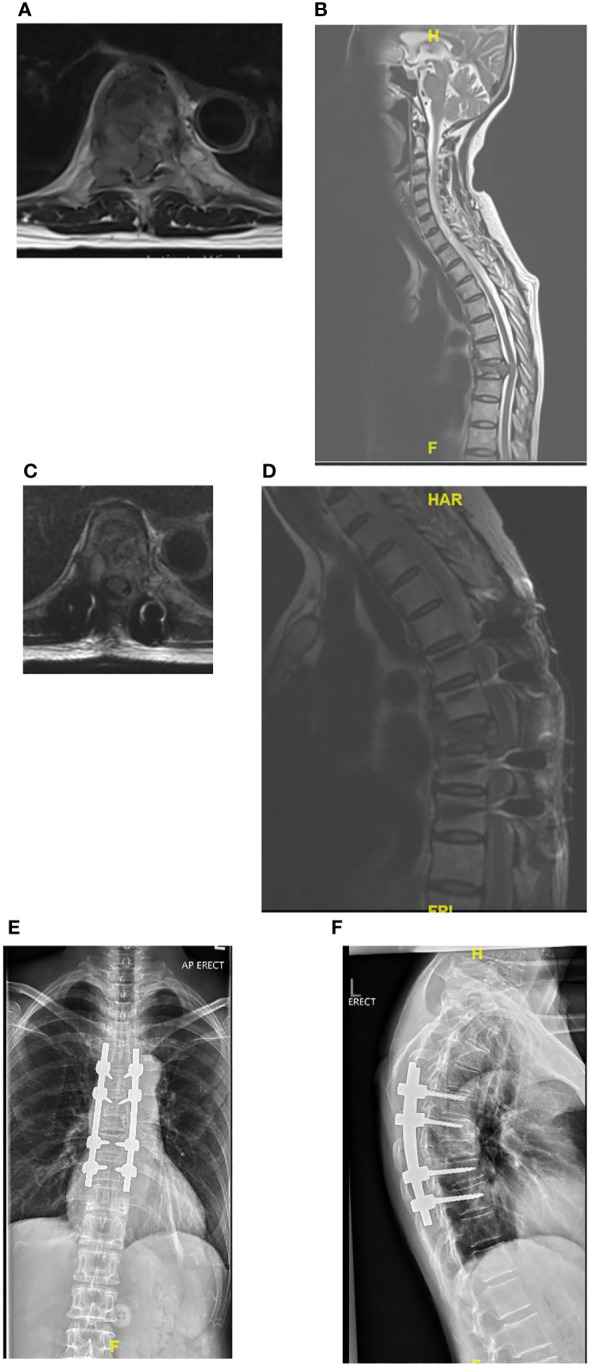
(A–F) Showing patient with Non-Small Cell Lung Cancer metastatic epidural spinal cord compression at T8 treated with separation surgery and no anterior reconstruction or fusion. Post-operative imaging at 2 years show no evidence of construct failure. (A) Pre-operative Axial MRI of T8 with Bilsky 2 cord compression. (B) Pre-operative T2 Sagittal MRI with cord compression at T8. (C) Post-operative Axial MRI of T8 showing decompression of the spinal cord. (D) Post-operative Sagittal MRI showing decompression at T8. (E) Post-operative AP radiograph at 2 years follow-up. (F) Post-operative lateral x-ray at 2 years.
